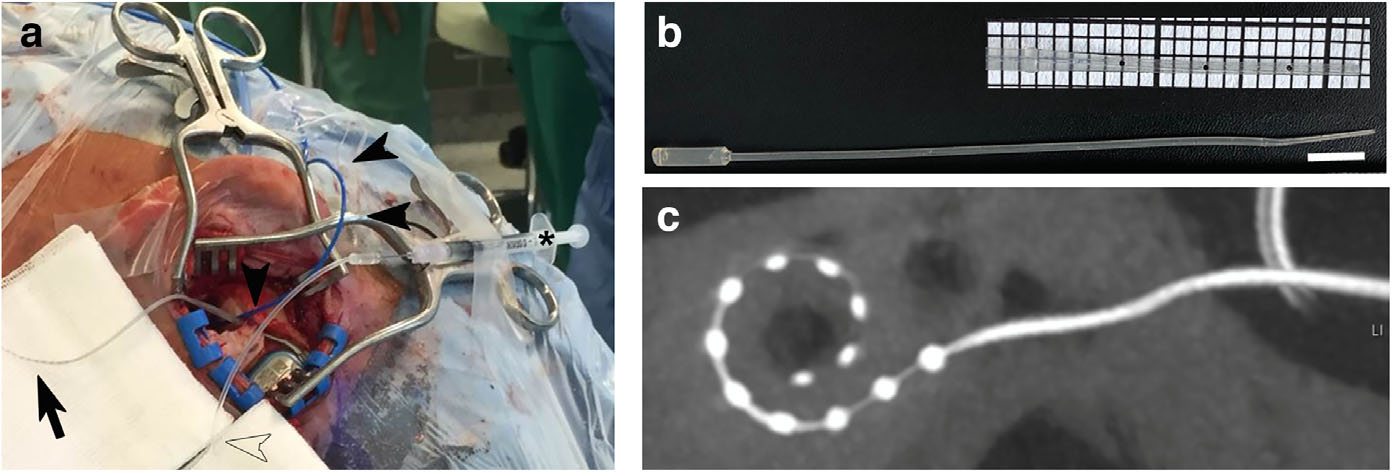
Surgical procedure and intraoperative UC‐MSC‐EV application. (a) Intraoperative image showing the mastoid with the electrode array prepared to be inserted (black bold arrow). Electrocochleography recordings were performed with an extra electrode (black arrow heads). The inner ear catheter (hollow arrow head) is attached to a syringe (asterisk) and contains the EV solution. (b) MED‐EL inner ear catheter with close up of the tip (insert). The tip has three marking spaced 5 mm apart to allow insertion to a predicted depth into the inner ear. Bar equals 1 cm. (c) Postoperative cone beam‐computed tomography showing the intracochlear position of the electrode array of the vesicle‐treated side
